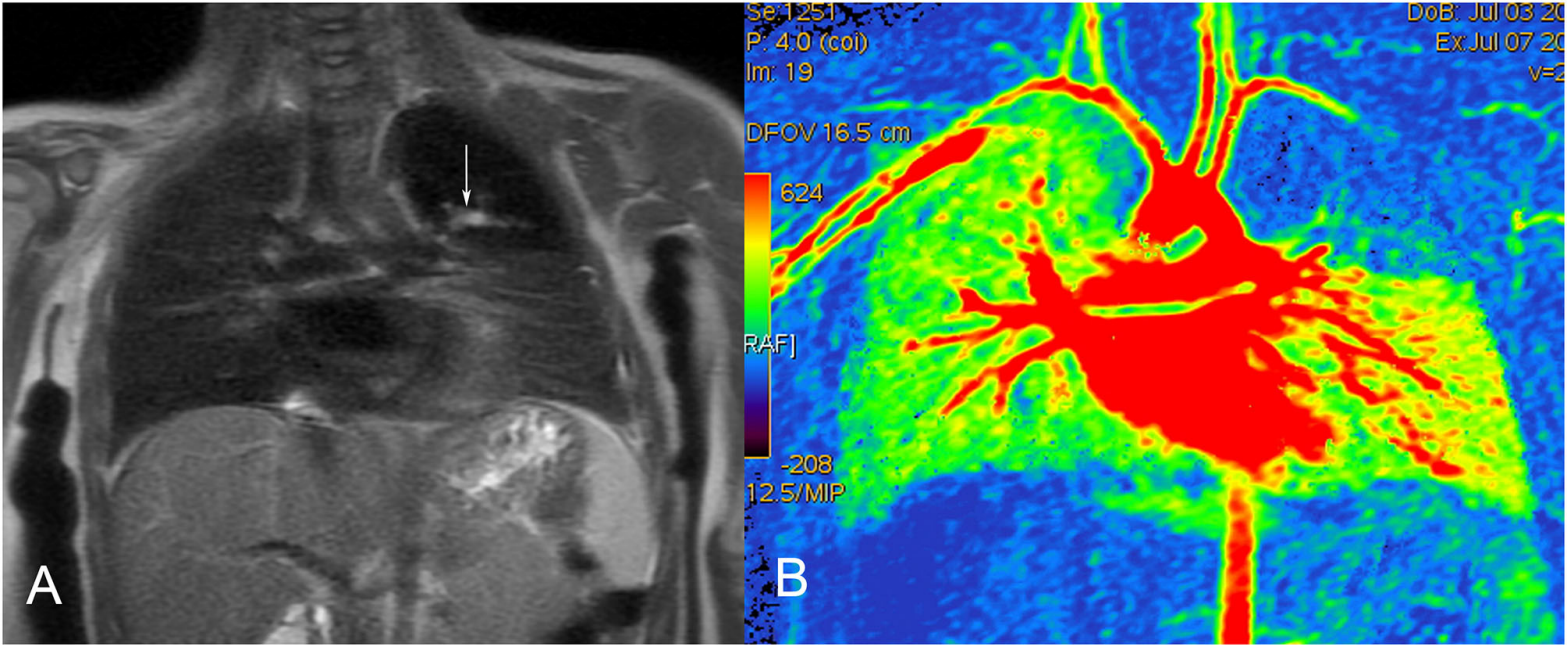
Bronchial atresia in a 12-month-old female. (A) MRI (T1-weighted coronal image) shows the mucocele as a branching hyperintense structure, extending into the abnormal left upper lobe. (B) Color-coded MRI perfusion image shows decreased flow to the affected lobe.
